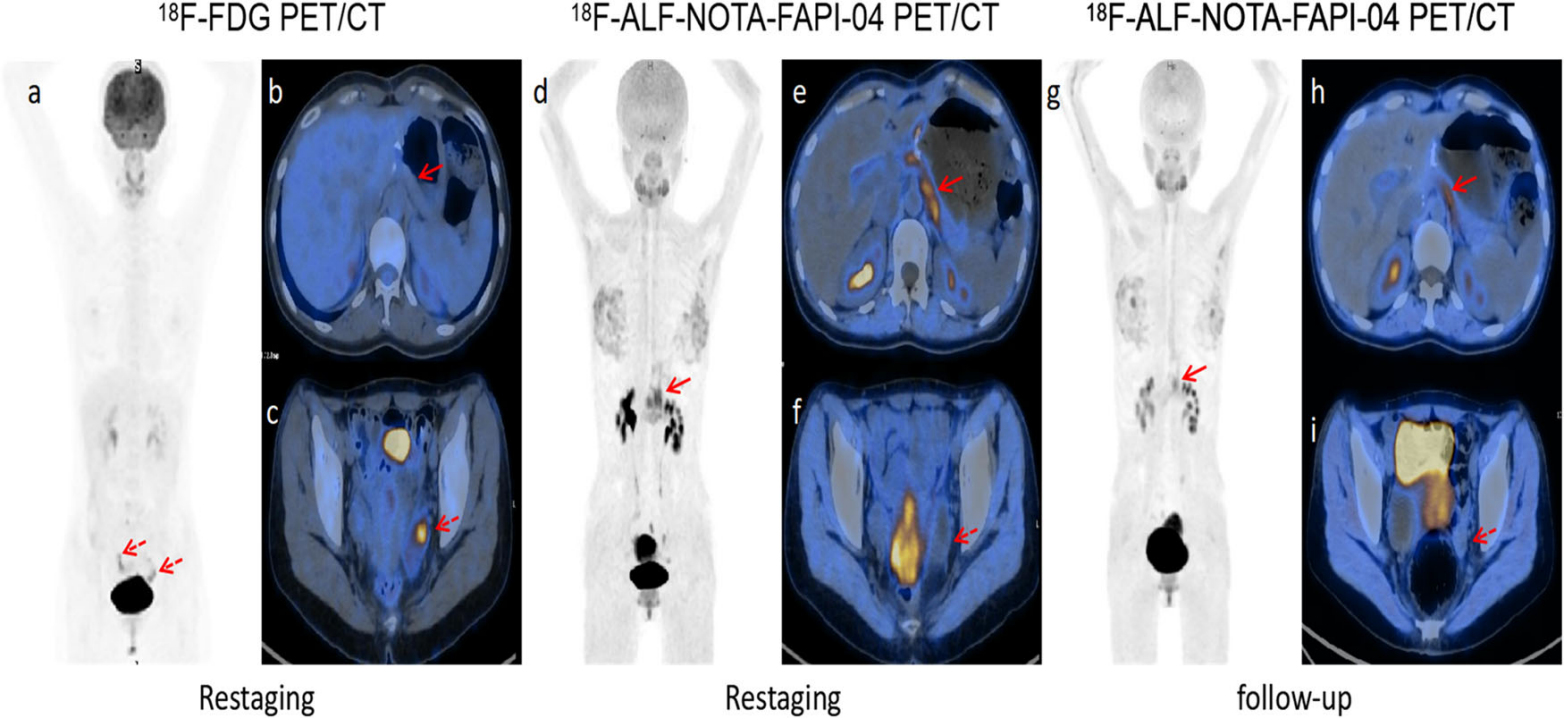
A 34-year-old female with a history of postoperative chemotherapy underwent PET/CT for restaging. Gastroscopy confirmed the recurrence of poorly differentiated adenocarcinoma containing SRCC in the gastric remnant. Restaging [^18^F]ALF-NOTA-FAPI-04 PET/CT (d, e) showed high uptake at the anastomotic site and residual gastric wall (solid arrows, SUVmax = 6.7), whereas [^18^F]FDG PET/CT (a, b) showed negative uptake (solid arrows, SUVmax = 1.2). The follow-up [^18^F]ALF-NOTA-FAPI-04 PET/CT (g, h) after 4 cycles of chemotherapy shows partial response(solid arrows, SUVmax = 3.9). [^18^F]FDG PET/CT (a, c) showed increased uptake in the ovaries bilaterally (dashed arrows, SUVmax = 10.8), but [^18^F]ALF-NOTA-FAPI-04 PET/CT (d, f, g, i) showed negative uptake (dashed arrows). After two years of follow-up CT, the lesion was confirmed to be physiologic uptake in the ovary
